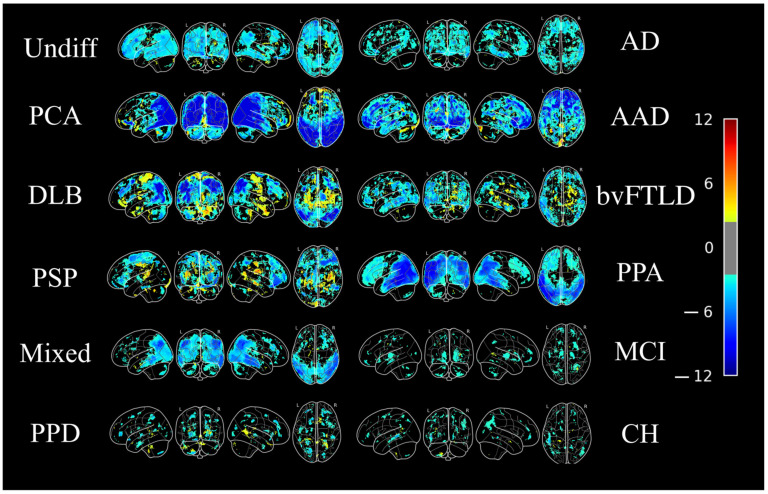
Averaged FDG-PET brain images of different types of dementia. The FDG-PET images have been z-scored to the mean and standard deviation of 111 cognitively healthy individuals that were included in the training set of MAD derivation in our previous study [44], then averaged within each dementia type. Undiff: Undifferentiated dementia; AD: Alzheimer’s disease; PCA: Posterior cortical atrophy; AAD: Atypical Alzheimer’s disease (frontal); DLB: Dementia with Lewy bodies; bvFTLD: Frontotemporal lobar degeneration, behavioural variant; PSP: Progressive supranuclear palsy; PPA: Primary progressive aphasia; Mixed: Mixed dementia (vascular + AD); MCI: Mild cognitive impairment; PPD: Primary psychiatric disorder; CH: Cognitively healthy. The colour bar represents *z* value.
